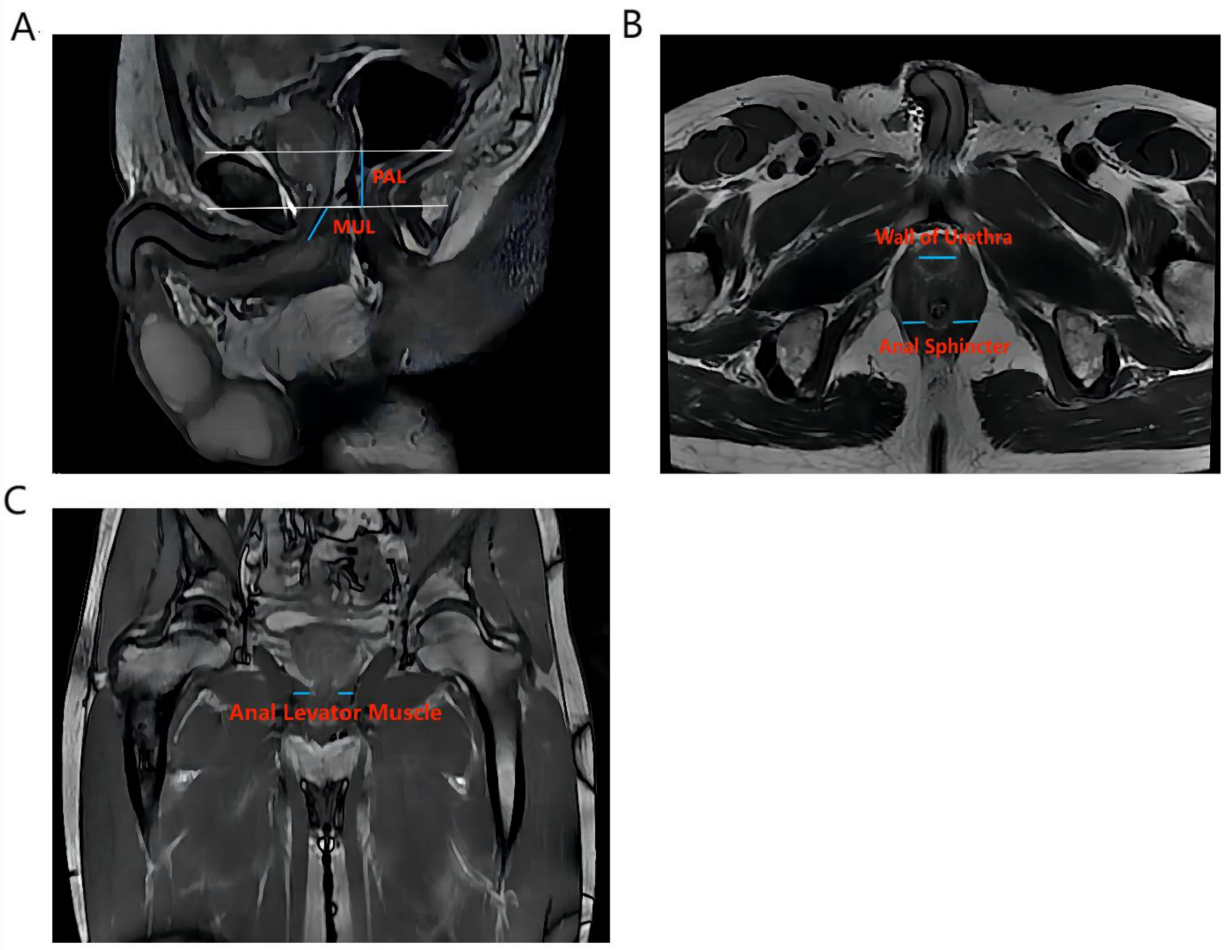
The main measurement indicators of anatomical structure on all T2-weighted images of patients. Figure 1A shows an example of a sagittal parameter on preoperative MR image; Figure 1B shows an example of a preoperative axial plane parameter; Figure 1C shows an example of a preoperative coronal parameter. PAL: pubic symphysis‒prostate apex length; MUL: membranous urethral length
